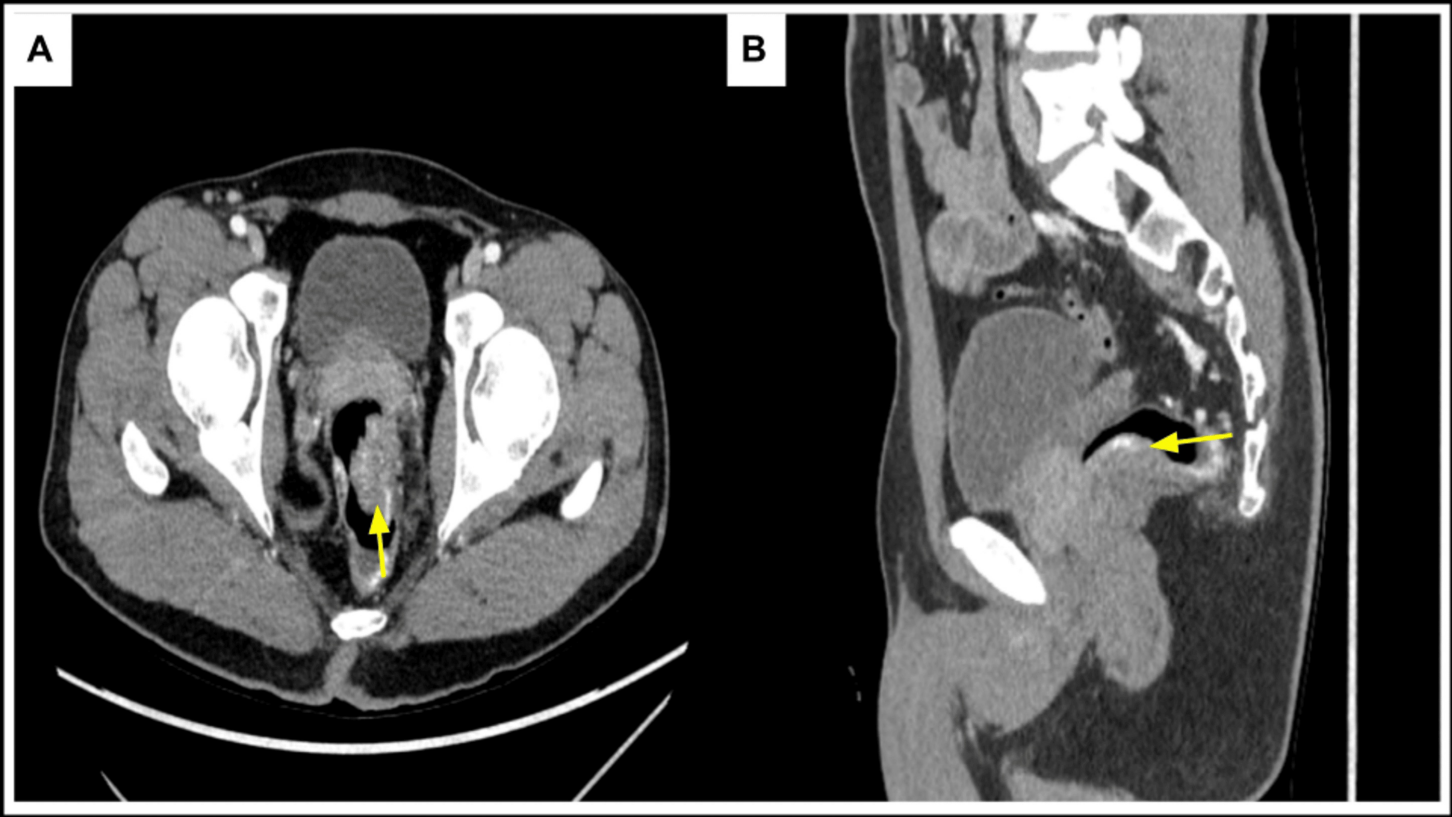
Computed tomography of the pelvis with intravenous contrast on portal venous phase demonstrates a heterogeneous enhancing endoluminal soft tissue lesion (yellow arrow) arising from the rectal wall viewed on axial (A) and sagittal (B) planes. This is consistent with biopsy-proven rectal schwannoma.
